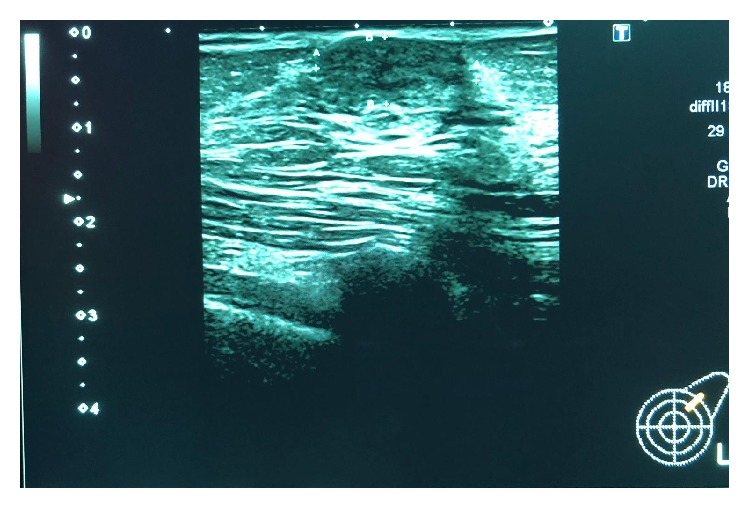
Ultrasound of the left breast showing a well-defined hypoechoic solid mass lesion, measuring 16 mm × 7 mm.
